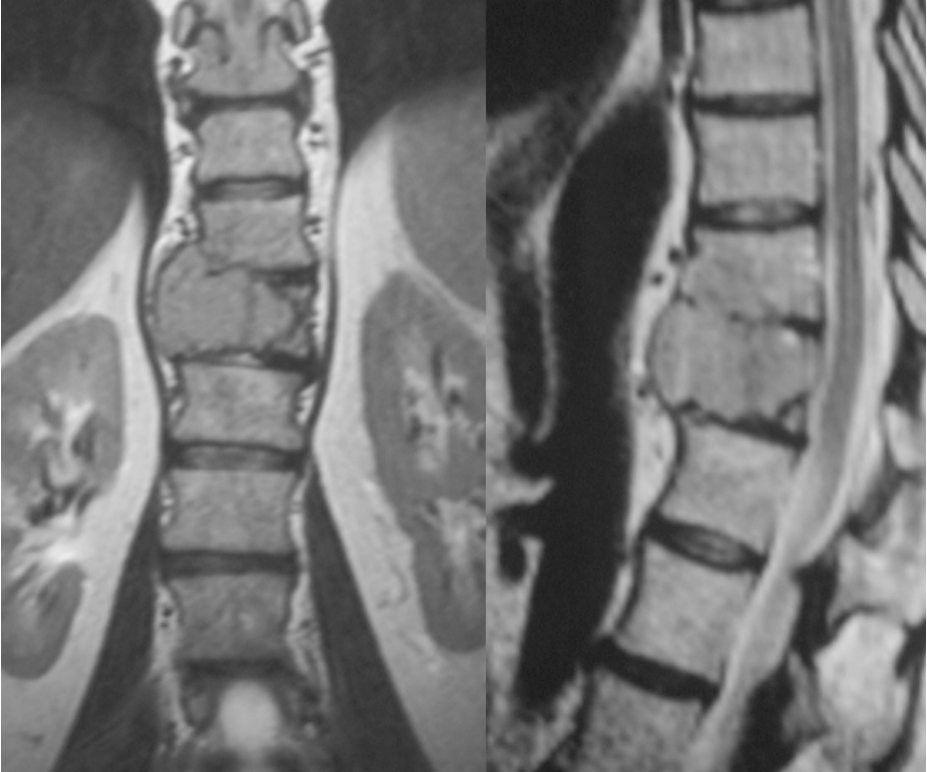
MRI of lesion. T2-weighted MRI image showing the tumour in D11 involving the upper vertebra as well.
